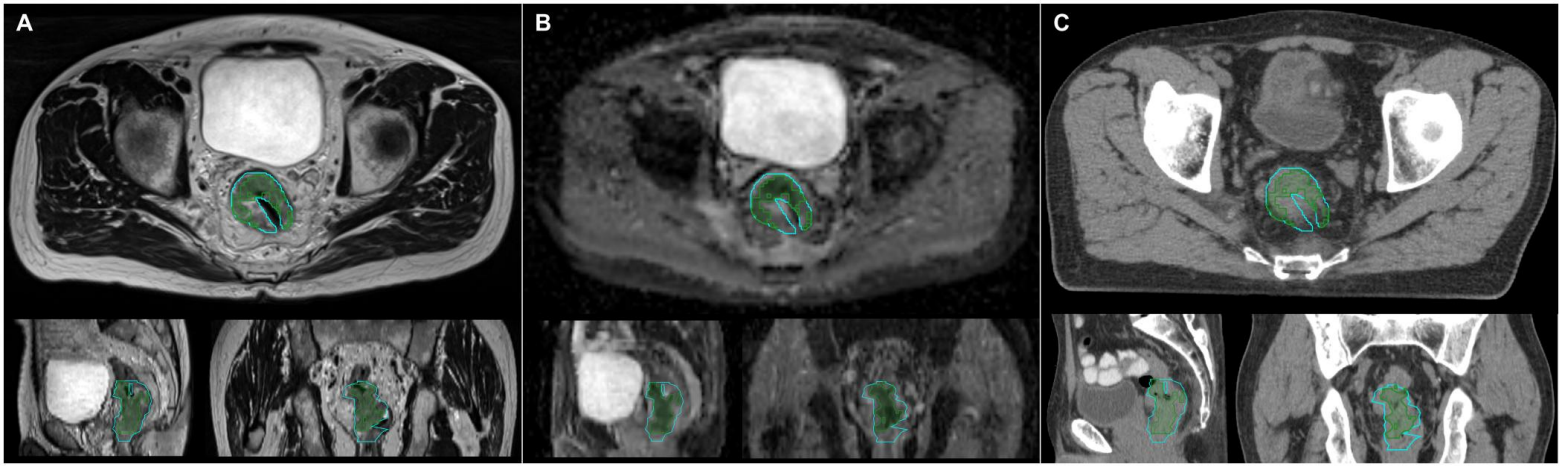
Example of GTV (cyan) and C40 (green) contours on (A) T2, (B) DWI, and (C) CT.
